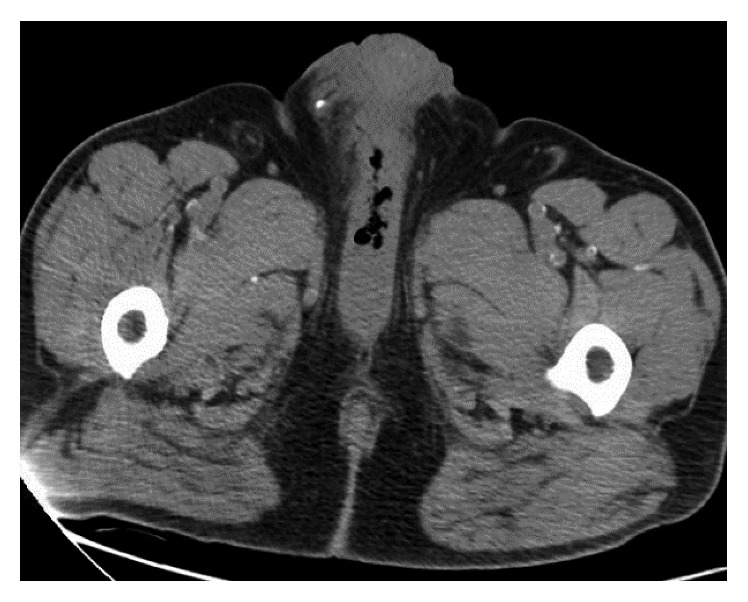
Axial view of pelvic CT image demonstrating emphysematous changes in the bulb of the corpus spongiosum.
